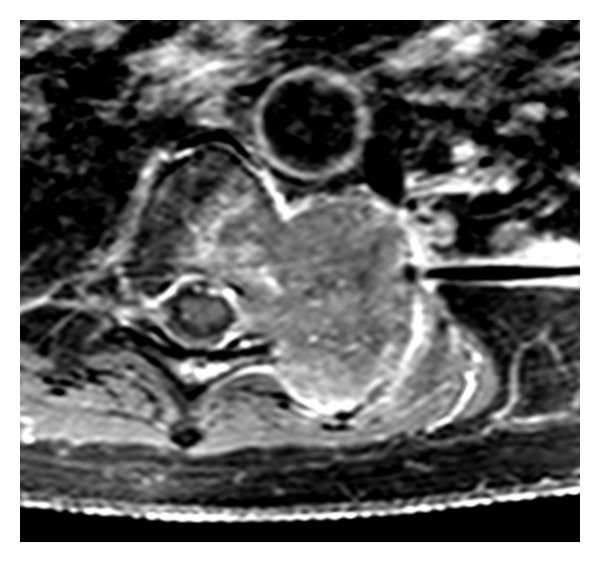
T1 post-Gadolinium fat-saturation MRI T-spine. Note the metastatic tumor in the left paravertebral/chest wall area extending into the epidural space with destruction of the left transverse processes, pedicles, and vertebral bodies. Mass measures 4.0 cm in width × 5.8 cm cephalocaudally × 4.3 cm anterior-posterior direction.
